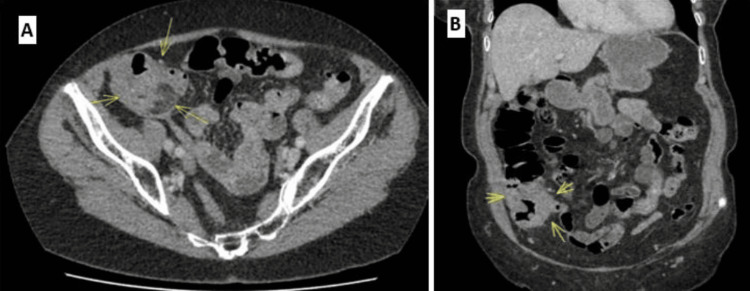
Contrast CT abdomen and pelvis (A) axial (B) coronal (area of suspicion marked by yellow arrows)
